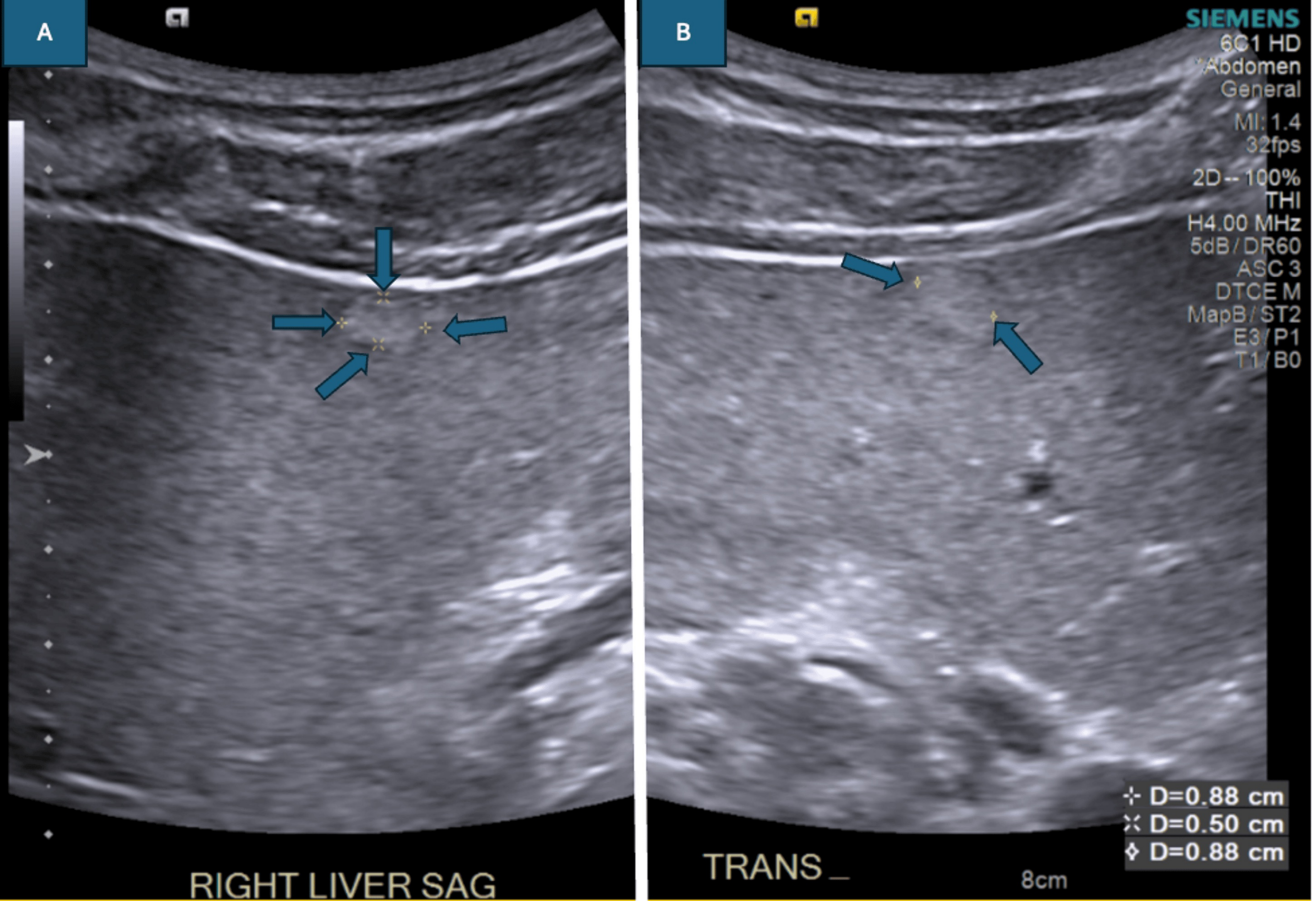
An ultrasound of the abdomen shows a measured lesion A. The hyperechoic right hepatic lesion measures a length of 9 mm and a width of 5 mm; B. The hyperechoic right hepatic lesion measures a height of 9 mm.
